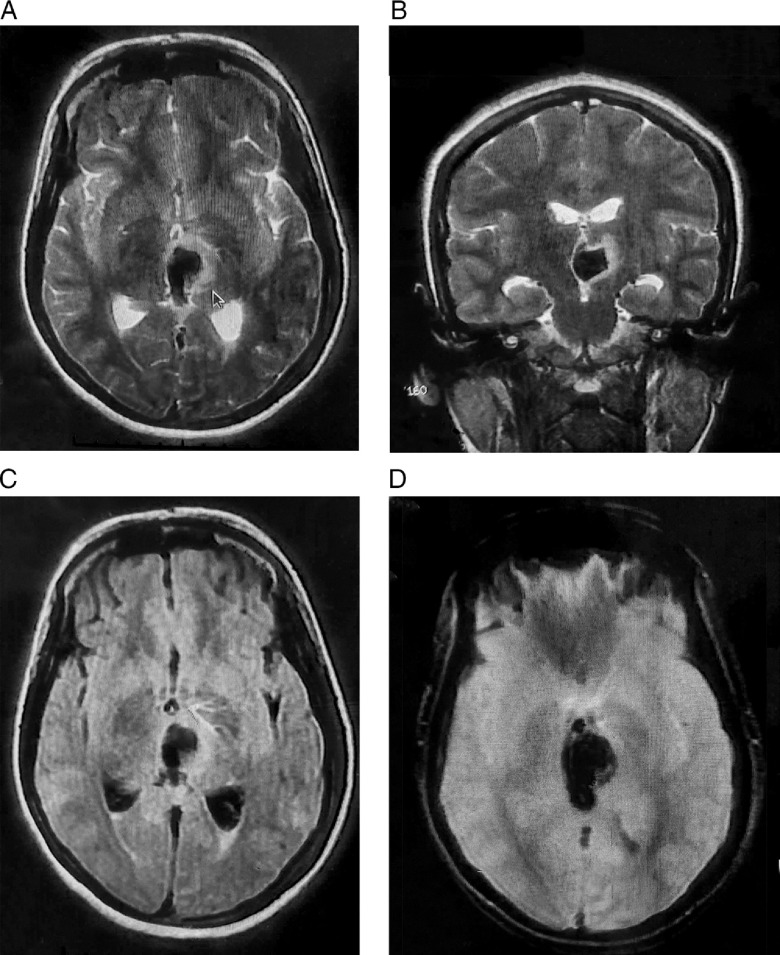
Contrast-enhanced magnetic resonance imaging of brain revealing non-enhancing focal altered intensity, which is hypointense in axial-T2 (A), coronal-T2 (B), and axial-fluid-attenuated inversion recovery (C) with signal blooming in axial-gradient echo sequences (D) at left thalamic area with perilesional edema and mass effect over third ventricle and associated intraventricular extension, suggestive of acute left thalamic intracerebral hemorrhage.
